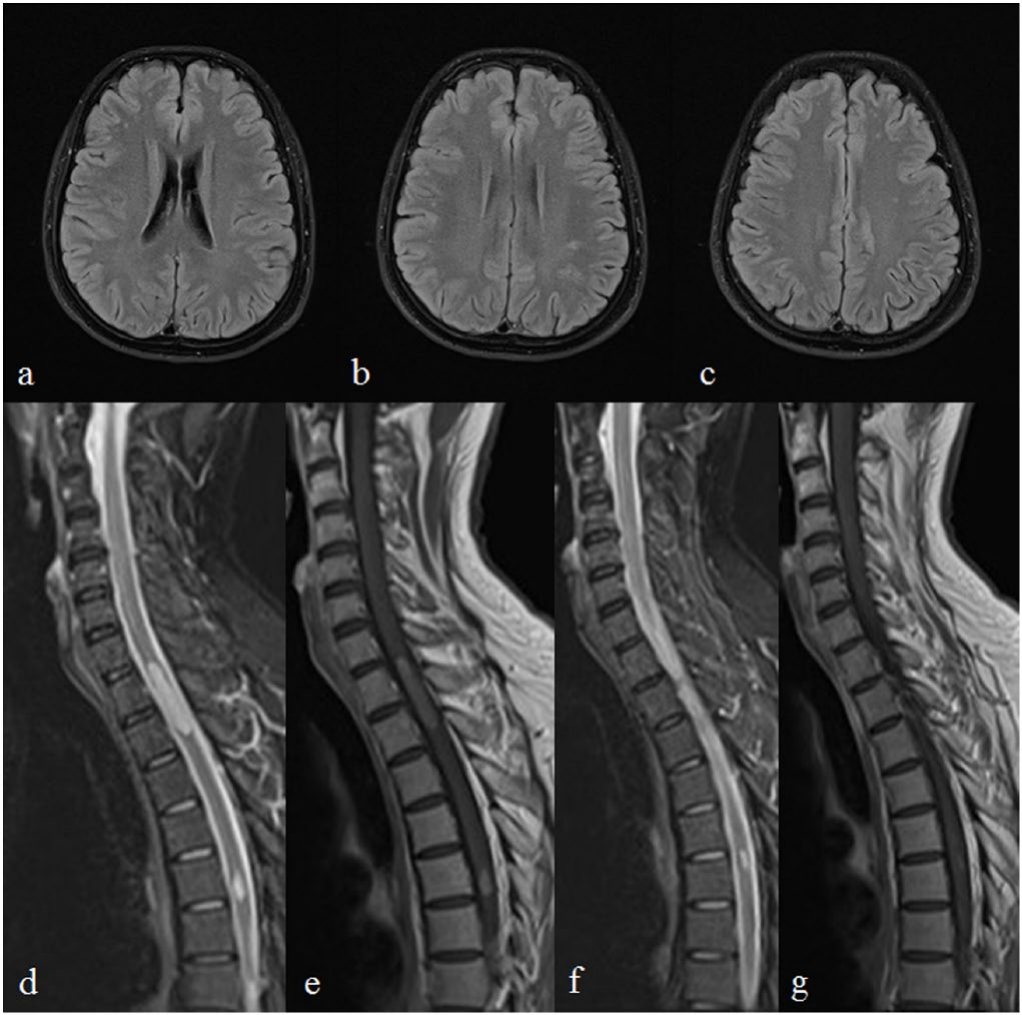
Brain and total spinal cord (SC) magnetic resonance imaging (MRI) performed
